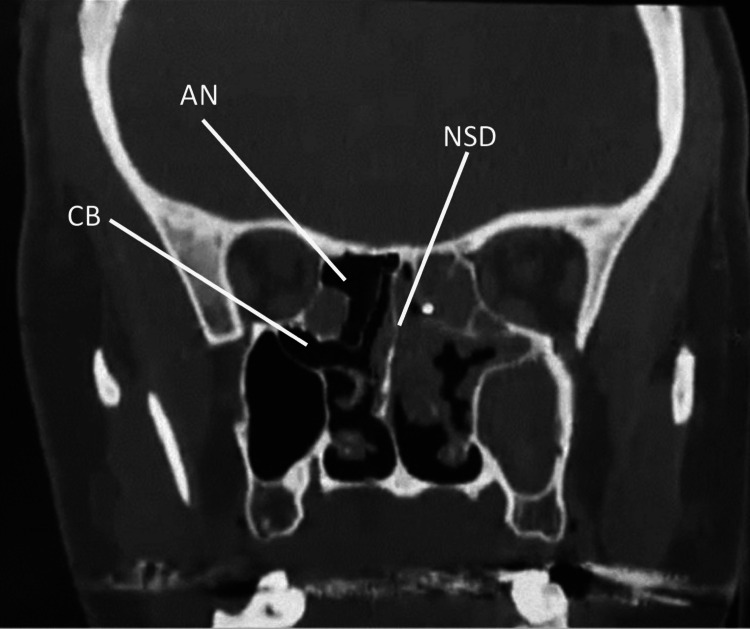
Coronal computed tomography (CT) scan of the paranasal sinuses demonstrating a cumulative anatomical variant burden within the ostiomeatal complex (OMC) White arrows indicate a prominent concha bullosa (CB), an agger nasi (AN) cell, and significant nasal septal deviation (NSD) to the opposite side, resulting in mechanical bottleneck obstruction and subsequent sinus opacification.
